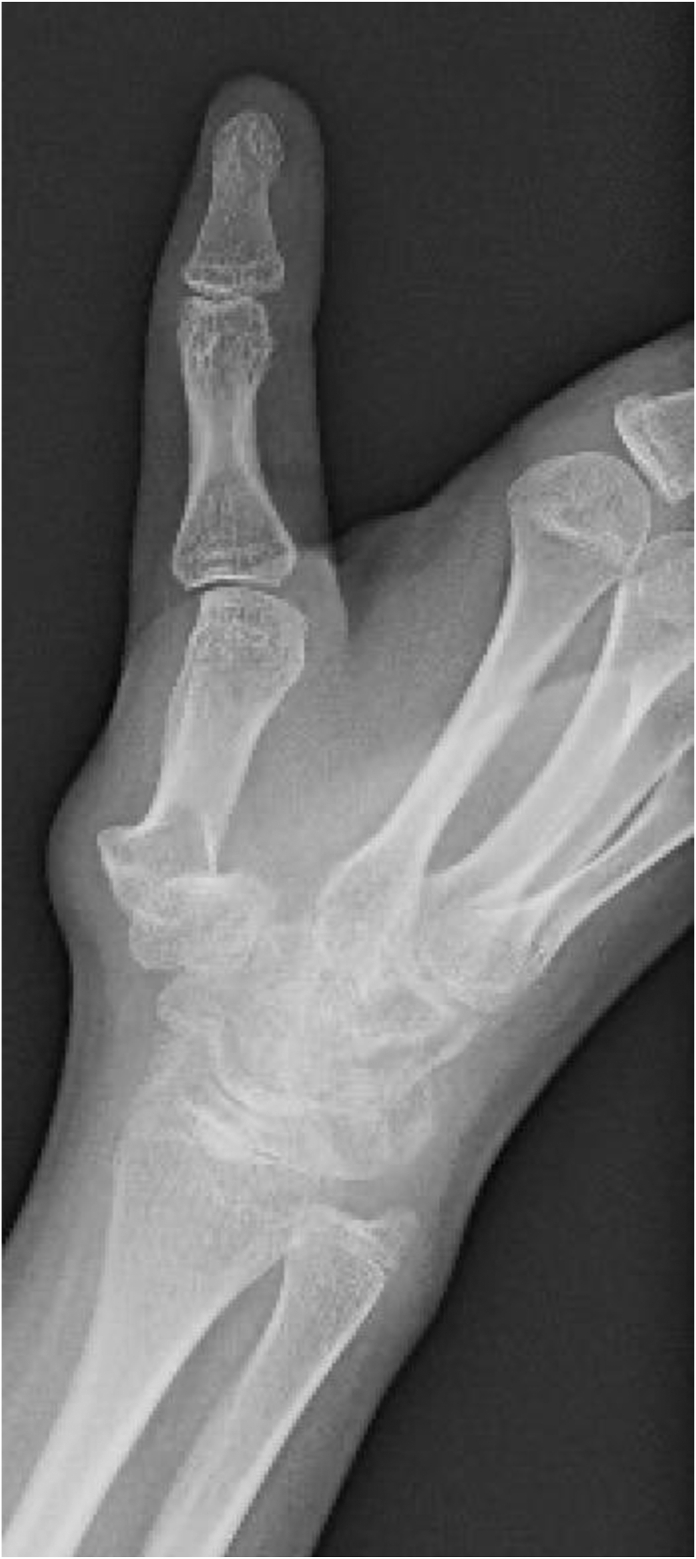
Anteroposterior radiograph of the right thumb demonstrating radial deviation of the thumb CMC and MCP joints.
